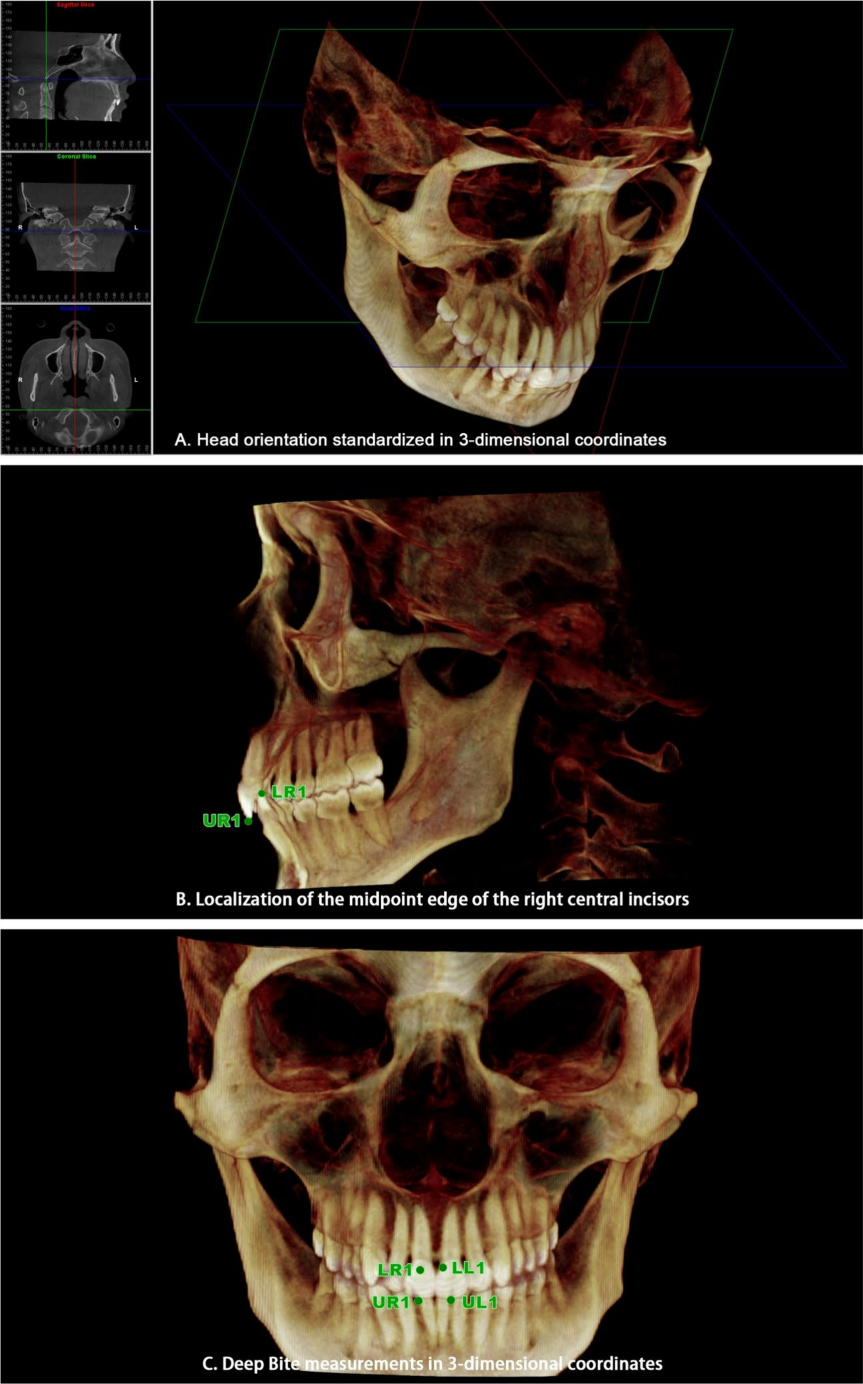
Head orientation and dental landmark identification in reconstructed three-dimensional (3D) cone-beam computed tomography images (CBCT). (A) Standardization of head orientation using three reference planes: the horizontal plane, midsagittal plane, and coronal plane. (B) Identification of the incisal edge midpoints of the upper right (UR1) and lower right (LR1) central incisors. (C) Visualization of all four dental landmarks: UR1, UL1 (upper left), LR1, and LL1 (lower left)
